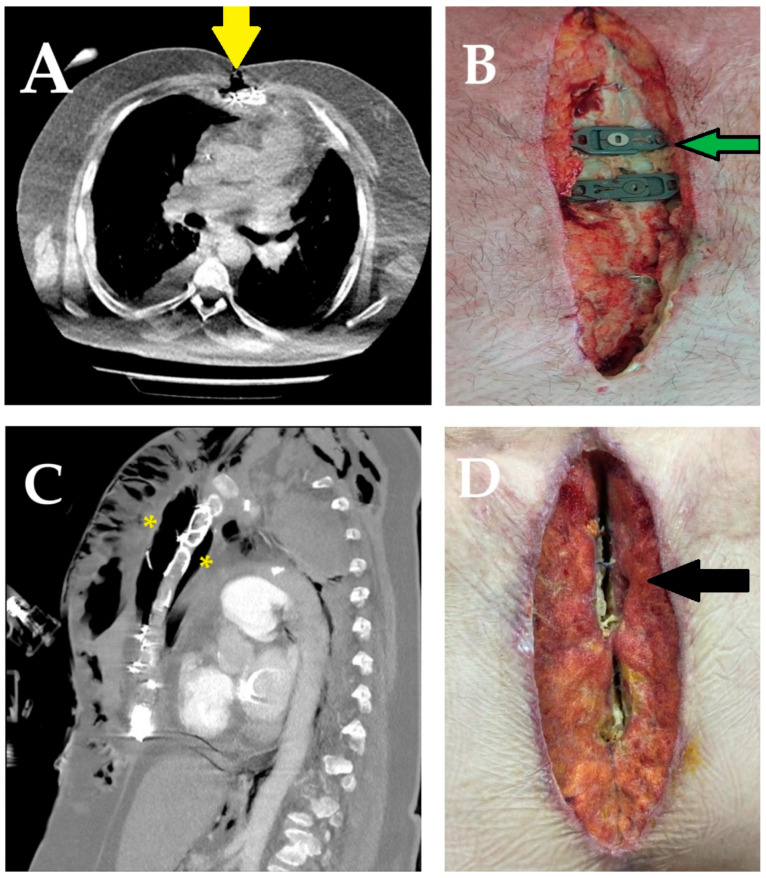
Representative cases of superficial and deep sternal wound infections. (A,B) An axial CT image of a 68-year-old obese, diabetic, and actively smoking male patient presenting with superficial wound drainage reveals subcutaneous air density anterior to the sternum (yellow arrow). During revision surgery, the incision had to be extended; the sternum and surrounding soft tissues were debrided, and a subpectoral muscle flap was used for reconstruction. The green arrow shows the sternal fixation material. (C,D) A sagittal CT view of a morbidly obese, diabetic female patient with deep sternal wound infection and dehiscence reveals extensive air accumulation both anterior and posterior to the sternum (yellow asterisks). During revision surgery, the sternum was found to be severely destructed (black arrow), and mediastinitis was treated with an omental flap.
